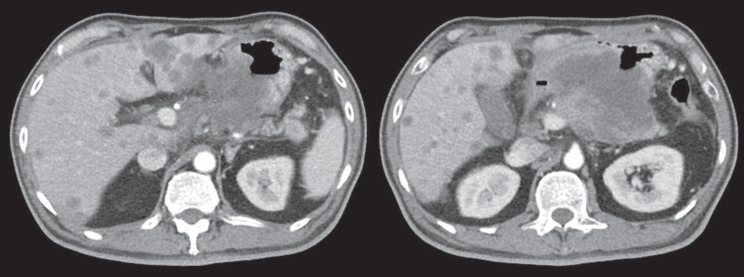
CT scan showing a heterogenous enhanced gastric mass abutting and probably invading the duodenum and pancreas with multiple metastatic lesions in the liver.
